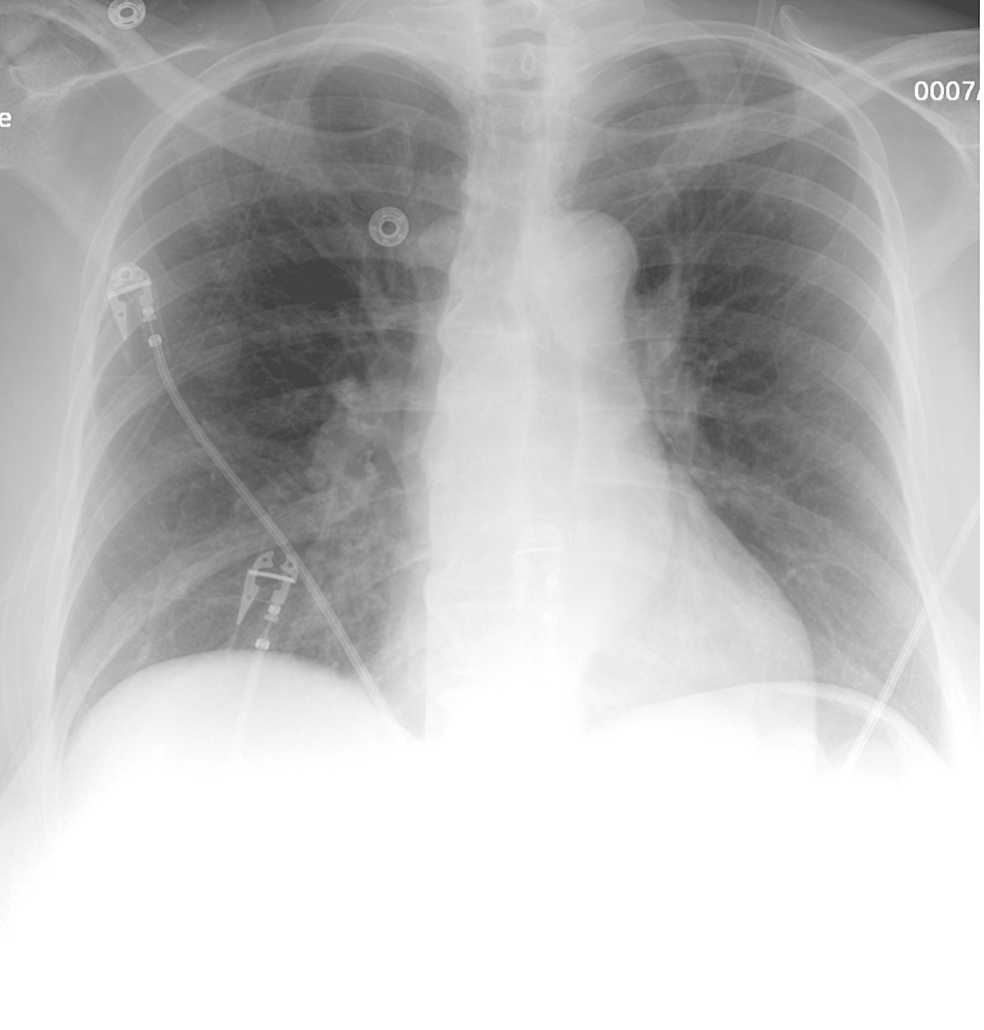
Chest X-ray with no radiographic evidence of an acute cardiopulmonary process
